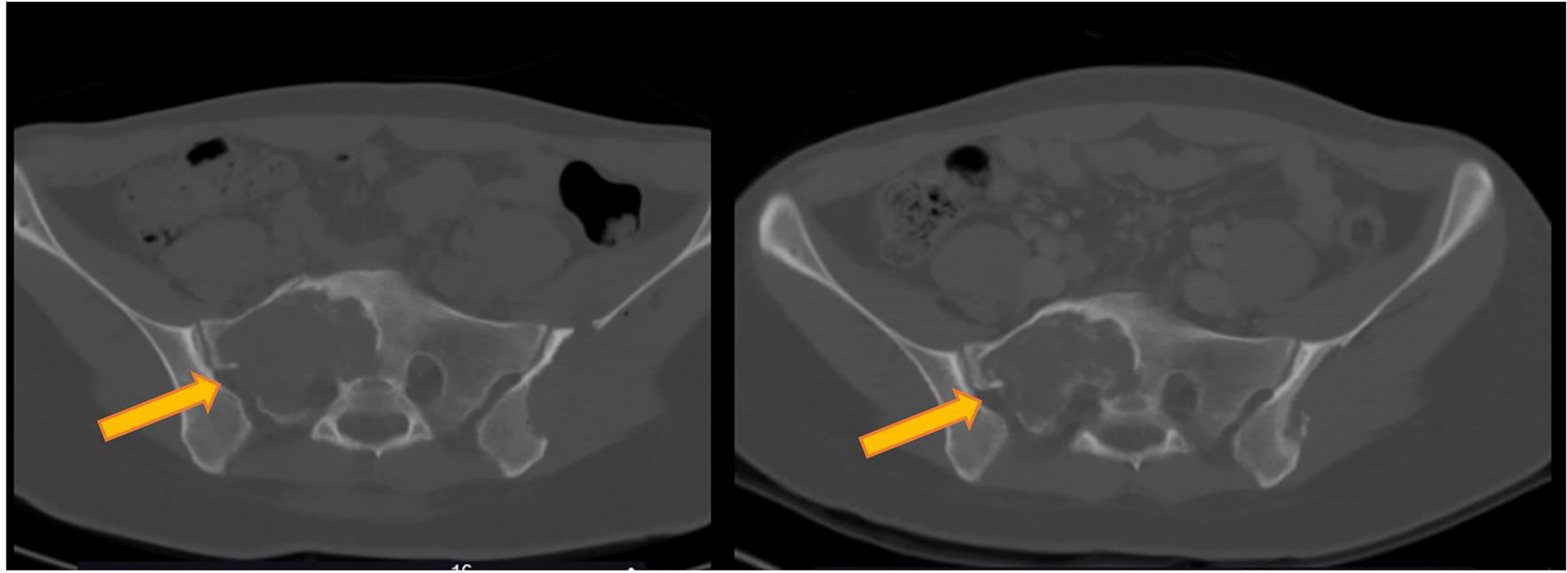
Response of target lesion (located in the sacrum) to denosumab in a metastatic giant cell tumor of the bone (GCTB). Left panel’s image is a CT scan showing a sacral lesion at baseline, and, on the right panel, a CT scan after 3 months of denosumab treatment showing a reduction in tumor size (partial response according to RECIST 1.1).
